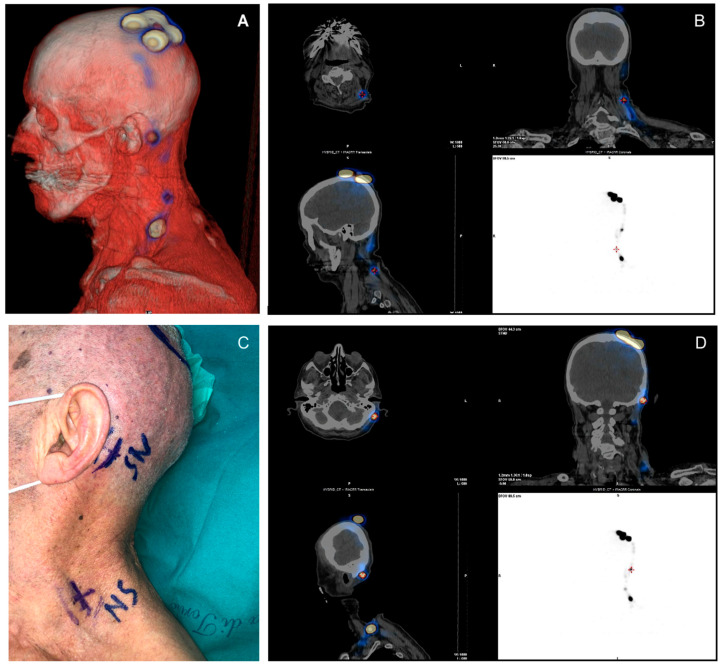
(Case 1): (A) A 3D image of the left retroauricular sentinel lymph node. (B–D) SPECT/CT rendering with a view of lymph nodes in CT reconstruction. (C) Preoperative image with skin marking of lymph nodes. SPECT-CT: single-photon emission computed tomography—computed tomography
